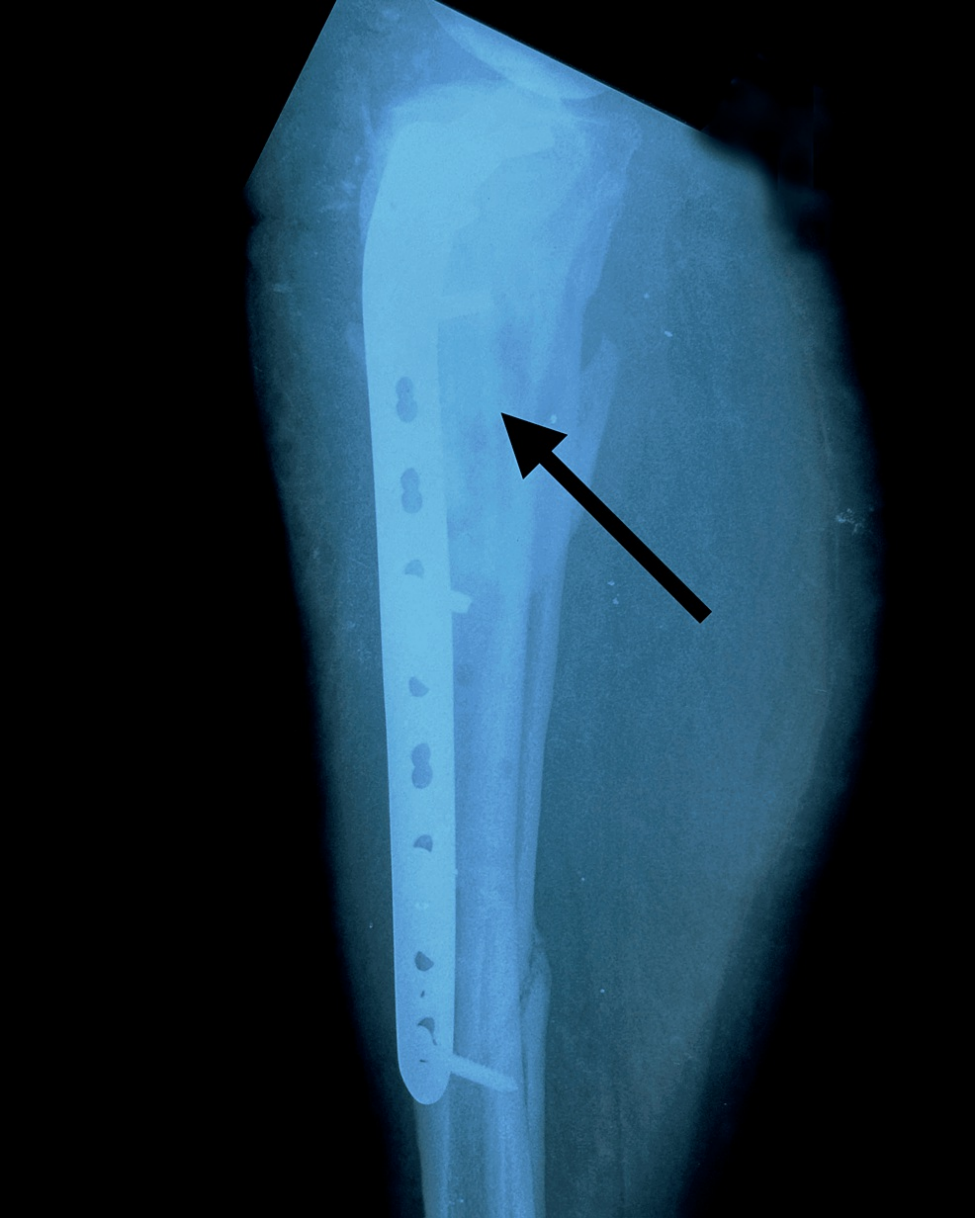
Postoperative lateral X-ray of the right leg showing fibular strut graft, proximal tibia locking plate and screws (arrow points to the fibula shaft strut autograft in the tibia medullary canal)
